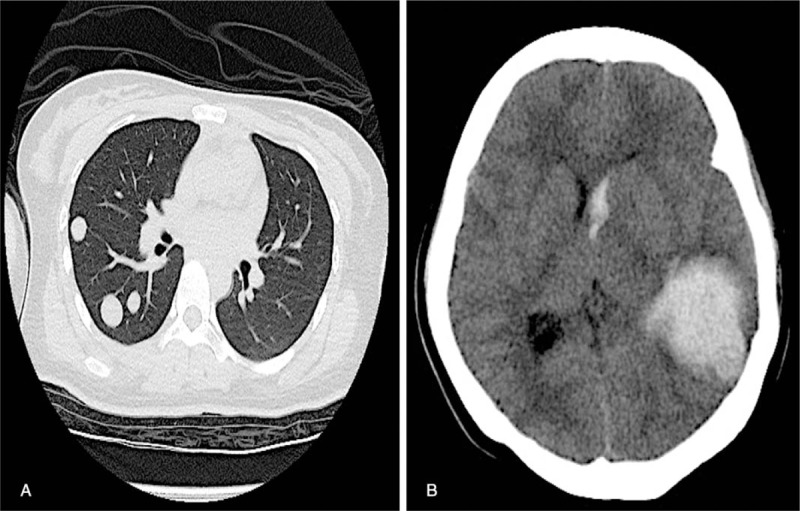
(A) Chest computed tomography (CT) scan carried out at 95 days postdelivery showing several metastatic nodules in the bilateral pulmonary tissue. (B) The cerebral CT carried out at 100 days postdelivery showing cerebral hemorrhage (left temporal lobe) and a midline shift of the brain.
